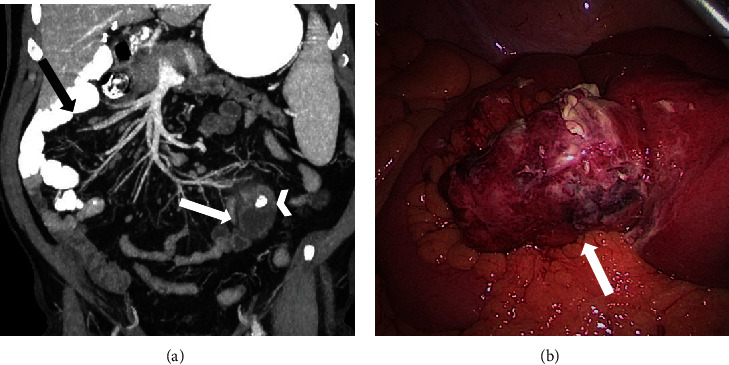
(a) Coronal reformatted contrast-enhanced CT images thickened with maximal intensity projection demonstrate malrotation of the small intestine with the jejunum and a large part of the ileum placed on the right side of the abdomen (black arrow). In the left hemiabdomen is a blind-ending tubular structure arising from the distal small bowel (white arrow), which has a markedly thickened wall with extensive surrounding fat stranding. Note the intraluminal calcified lesion (white arrowhead) contained in the saccular structure. (b) Laparoscopic view of perforated Meckel diverticulitis.
